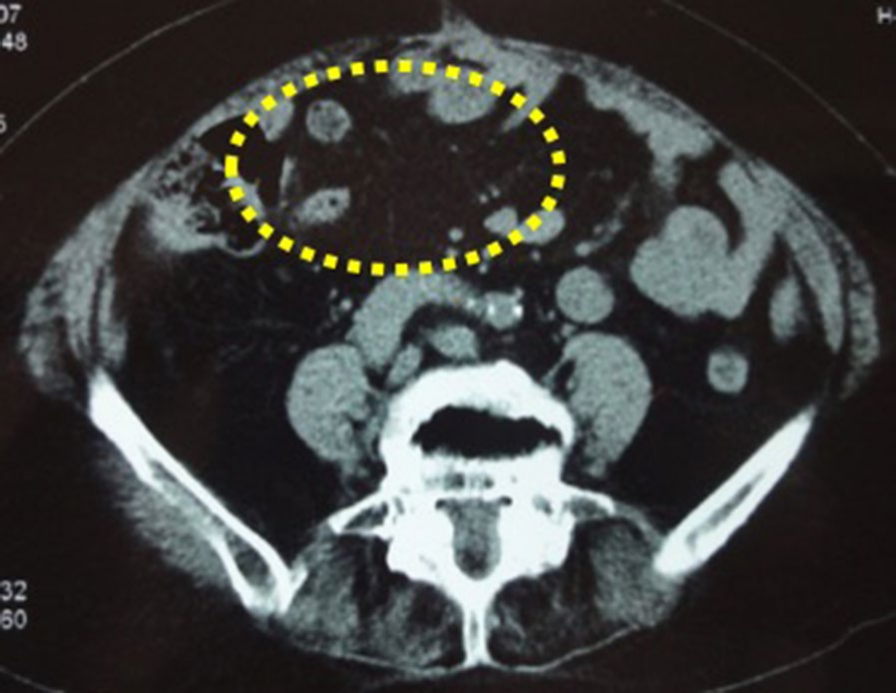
Complete recovery from intra-abdominal metastasis could be confirmed by an abdominal CT scan performed 1 year after endocrine therapy was started. Spotted circle (…) show the disappearance of intra-abdominal metastasis
